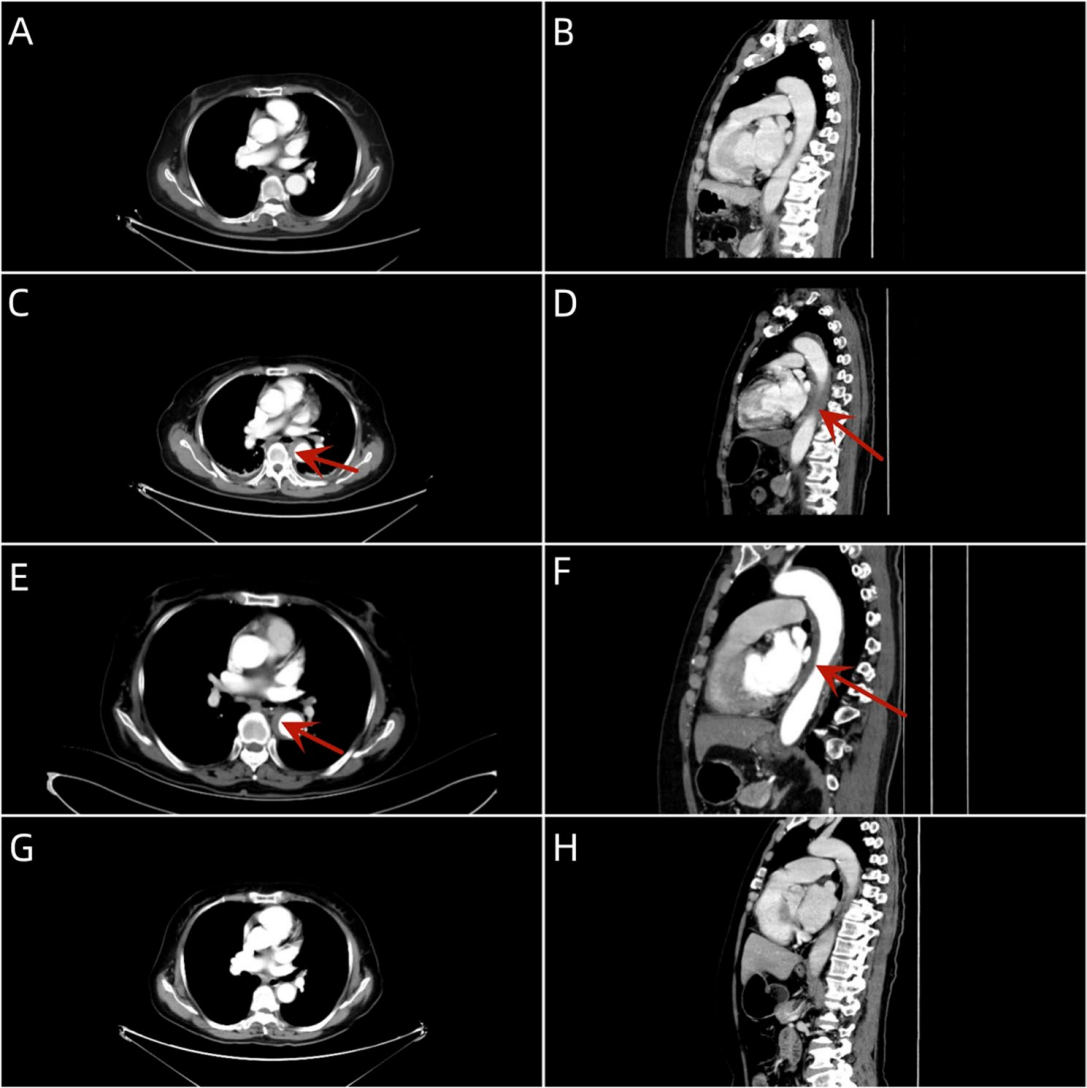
Chest computed tomography. *A: Preoperative axial CT image; B: Preoperative sagittal reconstructed CT image; C: Axial CT image at 7 h post-procedure; D: Sagittal reconstructed CT image at 7 h post-procedure; E: Axial MPR at postoperative day 7; F: Sagittal MPR image at postoperative day 7; G: Axial CT image at 10 months post-procedure; H: Sagittal reconstructed CT image at 10 months post-procedure; Red arrow denotes intramural hematoma location
